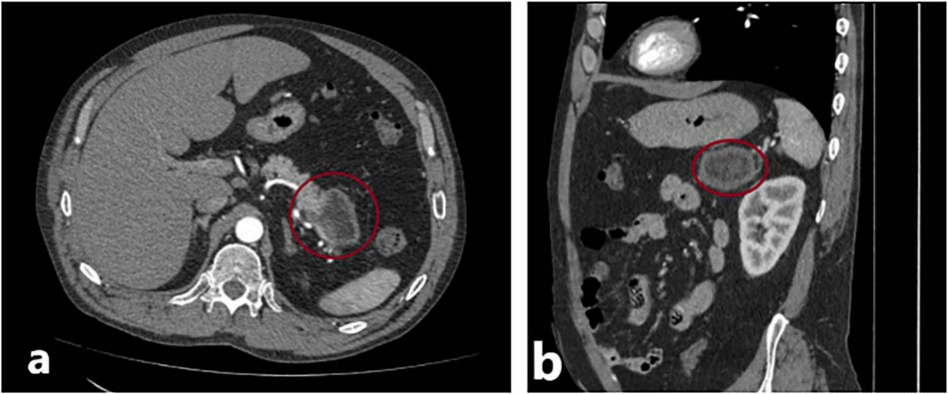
(a) and (b) Axial and sagittal CT images showing a distal pancreatic tumor.
